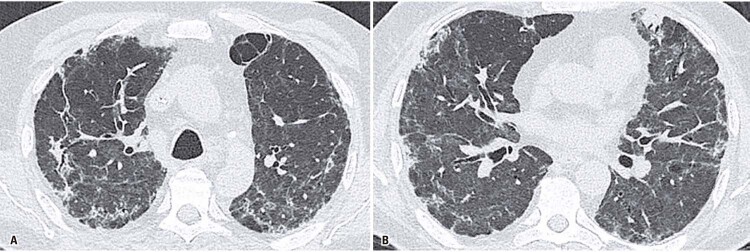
A and B) Fibrotic-like changes in a 62 years-old male patient, 92 days after COVID-19 initial symptoms onset, with abnormal diffusion capacity of carbon monoxide (53% of the predicted value). Chest computed tomography shows ground-glass opacities, linear and reticular abnormalities, discrete traction bronchiectasis and slight parenchymal architectural distortion
